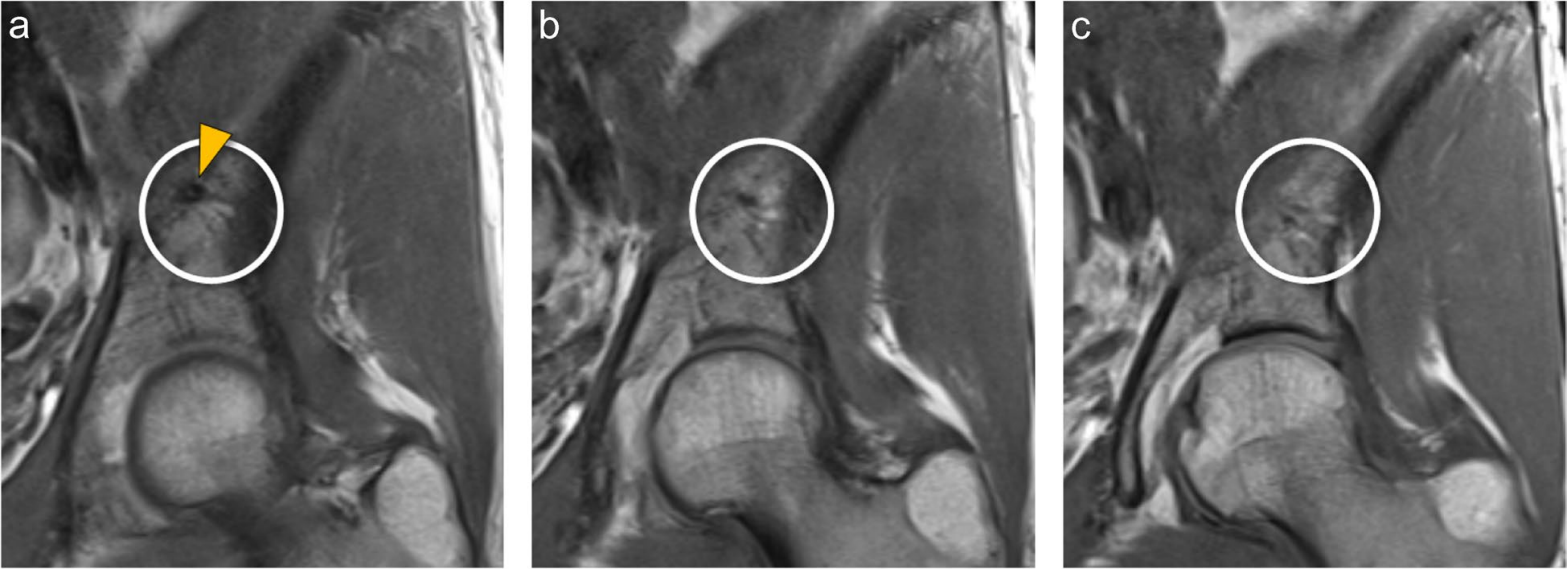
Central vessel convolute (CVC) in a 41-year-old female. Coronal T1-weighted MR images of the left ilium bone, shown from posterior to anterior. a Entry point of the iliolumbar artery (arrowhead) into the ilium bone. b, c The branches of the iliolumbar artery spread radially, forming the CVC (marked by the circle)
